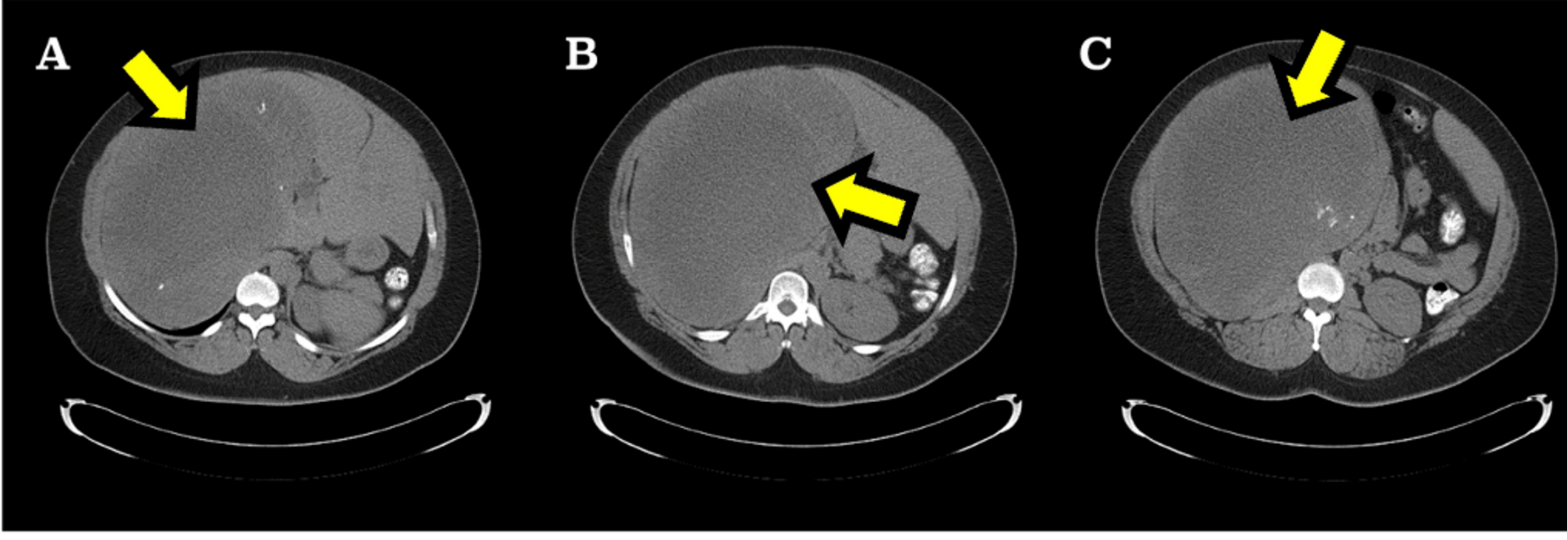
Contrast-enhanced abdominal computed tomography axial images (A-C). A large hypodense mass (arrows) is observed in the right hypochondrium, displacing the liver medially to the left.
